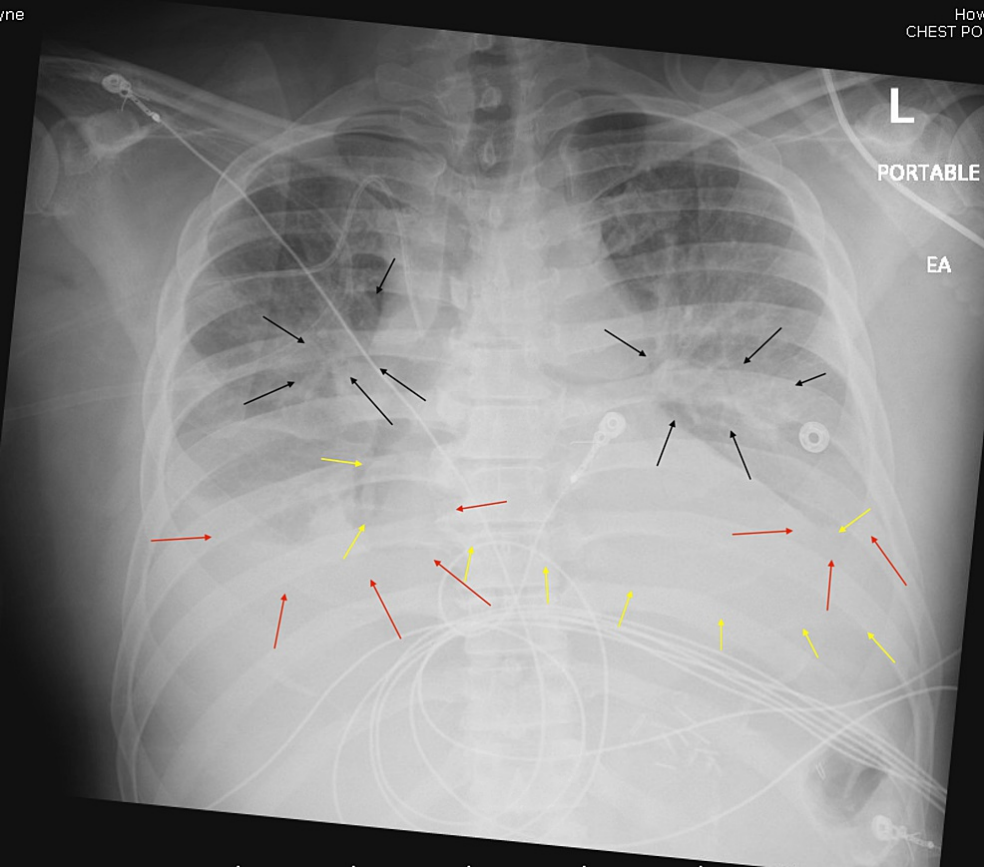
Chest X-ray on admission Black arrows: Pulmonary venous congestion, Red arrows: Bilateral pleural effusions, Yellow arrows: Enlarged cardiac silhouette
